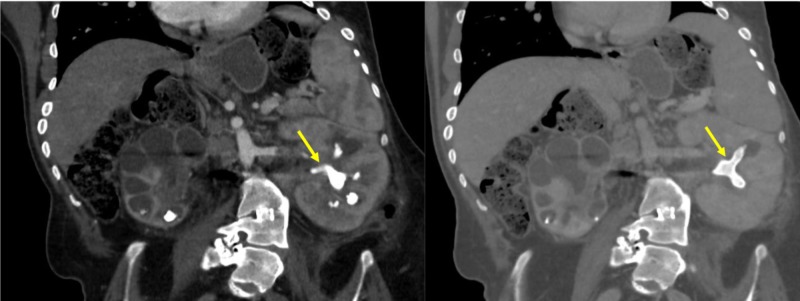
The left and right contrast-enhanced coronal computed tomography (CT) images show a large staghorn calculus in the left kidney (yellow arrow)
